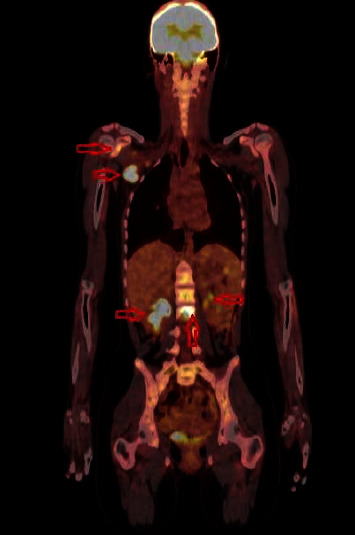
Positron-emission tomography/computed tomography: hypermetabolic involvement in the neck of the right scapula, T10 L2 vertebrae, hypermetabolic conglomerated lymphadenopathies in the right axillary region, hypodense hypermetabolic regions in the liver anterior of segments 5-6, and increased focal activity in the splenic hilus (red arrows).
